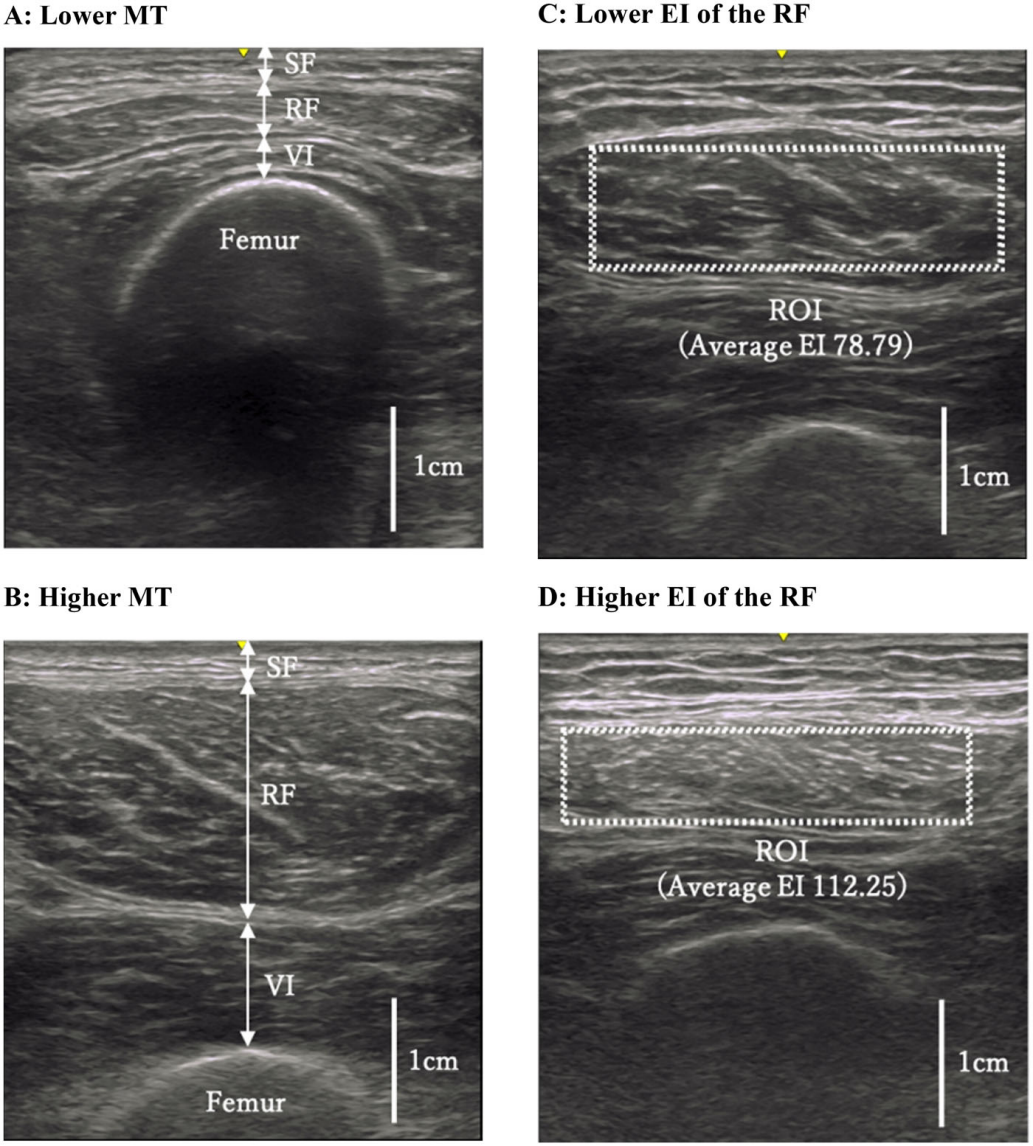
Ultrasound images of the quadriceps. (a,b) Thicknesses of the subcutaneous fat (SF), rectus femoris (RF) and vastus intermedius (VI) were measured at the position perpendicular to the femur in each image. (c,d) Dotted rectangle indicates maximum region of interest (ROI) in the RF. All images in Figure 1 were derived from our data, and have not been published elsewhere.
